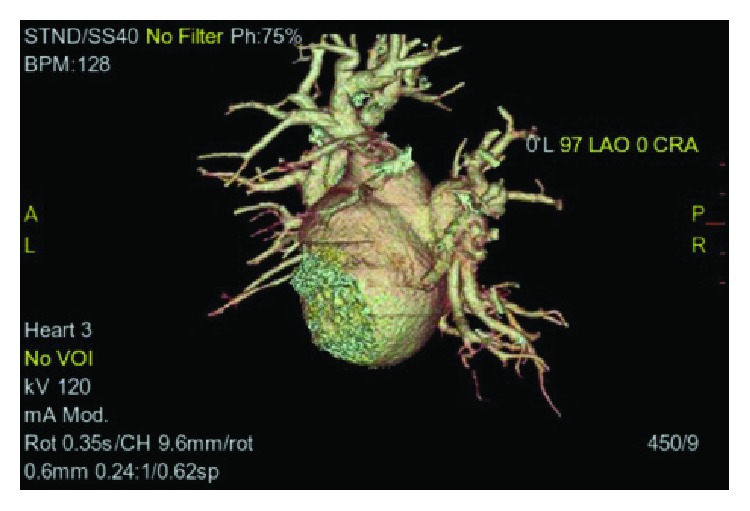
CTA of the pulmonary veins at 97 degrees steep LAO view showing there is no left atrial appendage on the heart surface.
